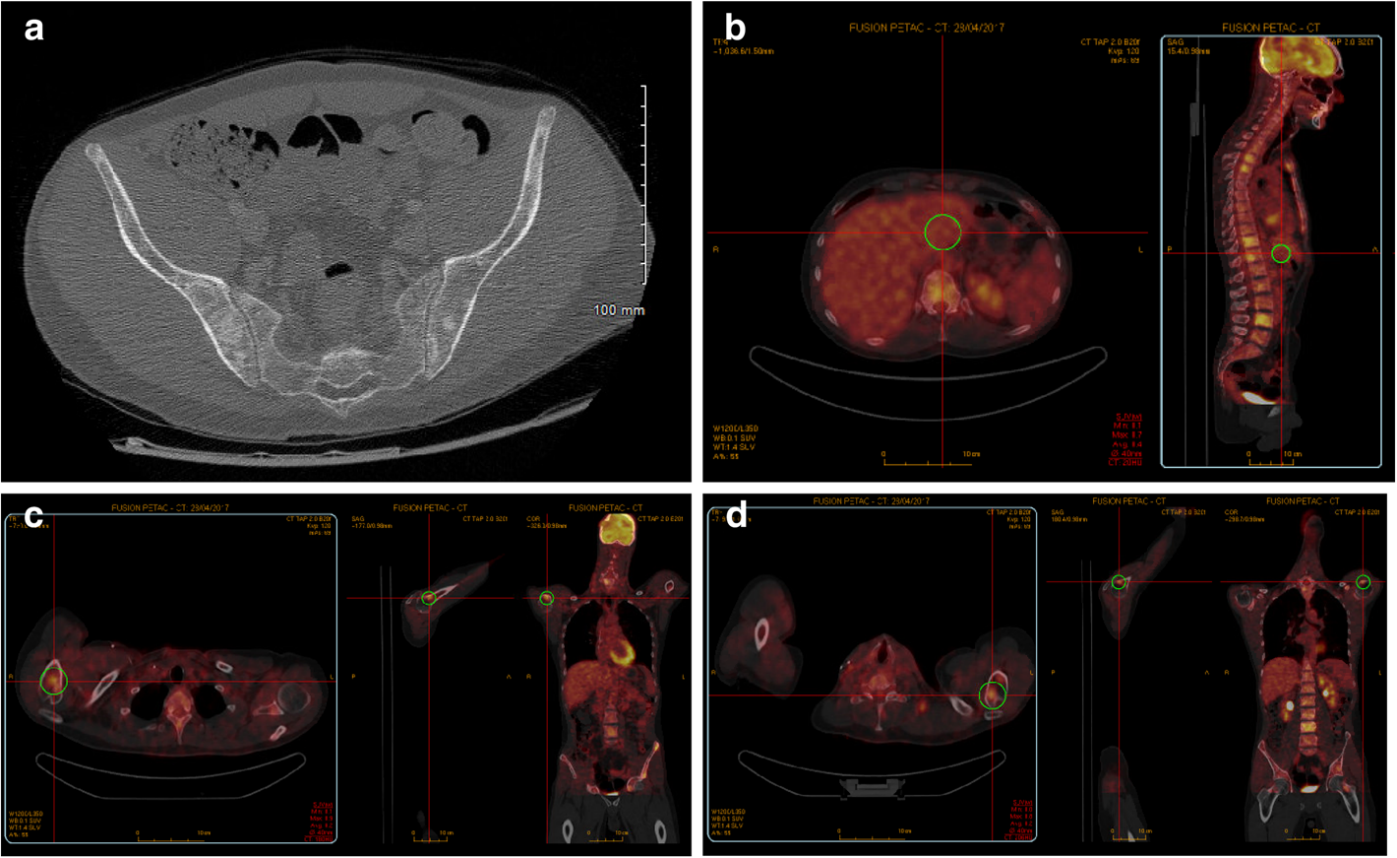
A computed tomography scan of the pelvis shows multiple osteoblastic bone lesions (a). Positon emission tomography-computed tomography scan demonstrates the hypermetabolic nature of these spinal bone lesions (b) located beyond the axial skeleton, shown here on the right scapula (c) and the left humerus (d)
